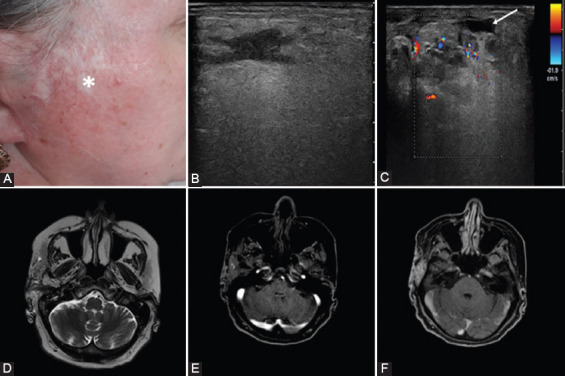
A 52-year-old woman with painful swelling at the level of the right parotid diagnosed with high-flow vascular malformation. (A) At clinical examination, atrophic changes of the skin were noted (*). (B) Transverse gray-scale sonogram shows an irregular hypoechoic lesion that extends into surrounding soft tissues which present edema. (C) On color Doppler sonogram, the lesion is composed of multiple abnormal vessels. Notice the presence of a superficial thrombosed vein (arrow). (D) Axial T2W1 shows a lobulated soft-tissue lesion with heterogeneous signal on T2WI. (E) Axial gadolinium-enhanced fat-suppressed T1WI demonstrates the presence of multiple vessels, some with early enhancement (5 s after contrast material injection) – arteries with origin in the right external carotid artery and (F) some vessels with late enhancement (70 s after contrast material injection).
